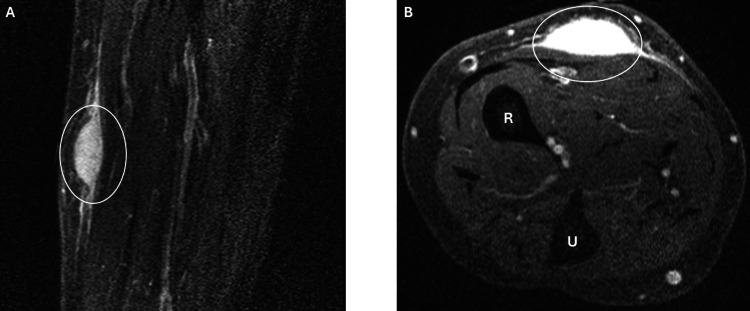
Magnetic resonance imaging (MRI) of the right radius/ulna A 1.9 cm diffuse enhancing ovoid soft tissue mass within the volar radial mid right forearm subcutaneous tissues, concerning for peripheral nerve sheath tumor of the peripheral nerve branch, sarcoma, or malignant fibrous histiocytoma. A) T1-weighted sagittal ovoid soft tissue mass (white circle); B) T1-weighted axial ovoid soft tissue mass (white circle), radius (R), and ulna (U).
